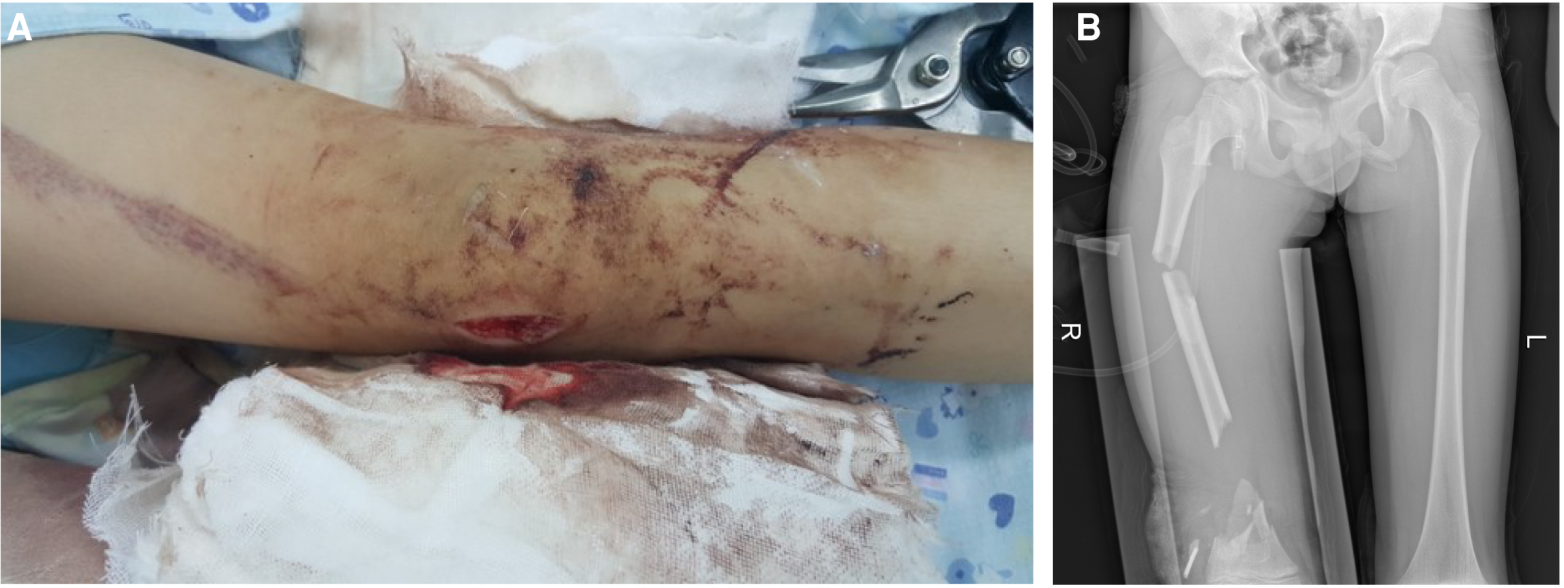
(A) A 4 cm open wound is visible at the distal end of the right femur. (B) Shows a plain radiograph of the femur, demonstrating a segmental fracture with significant bone loss in the right femoral diaphysis.
